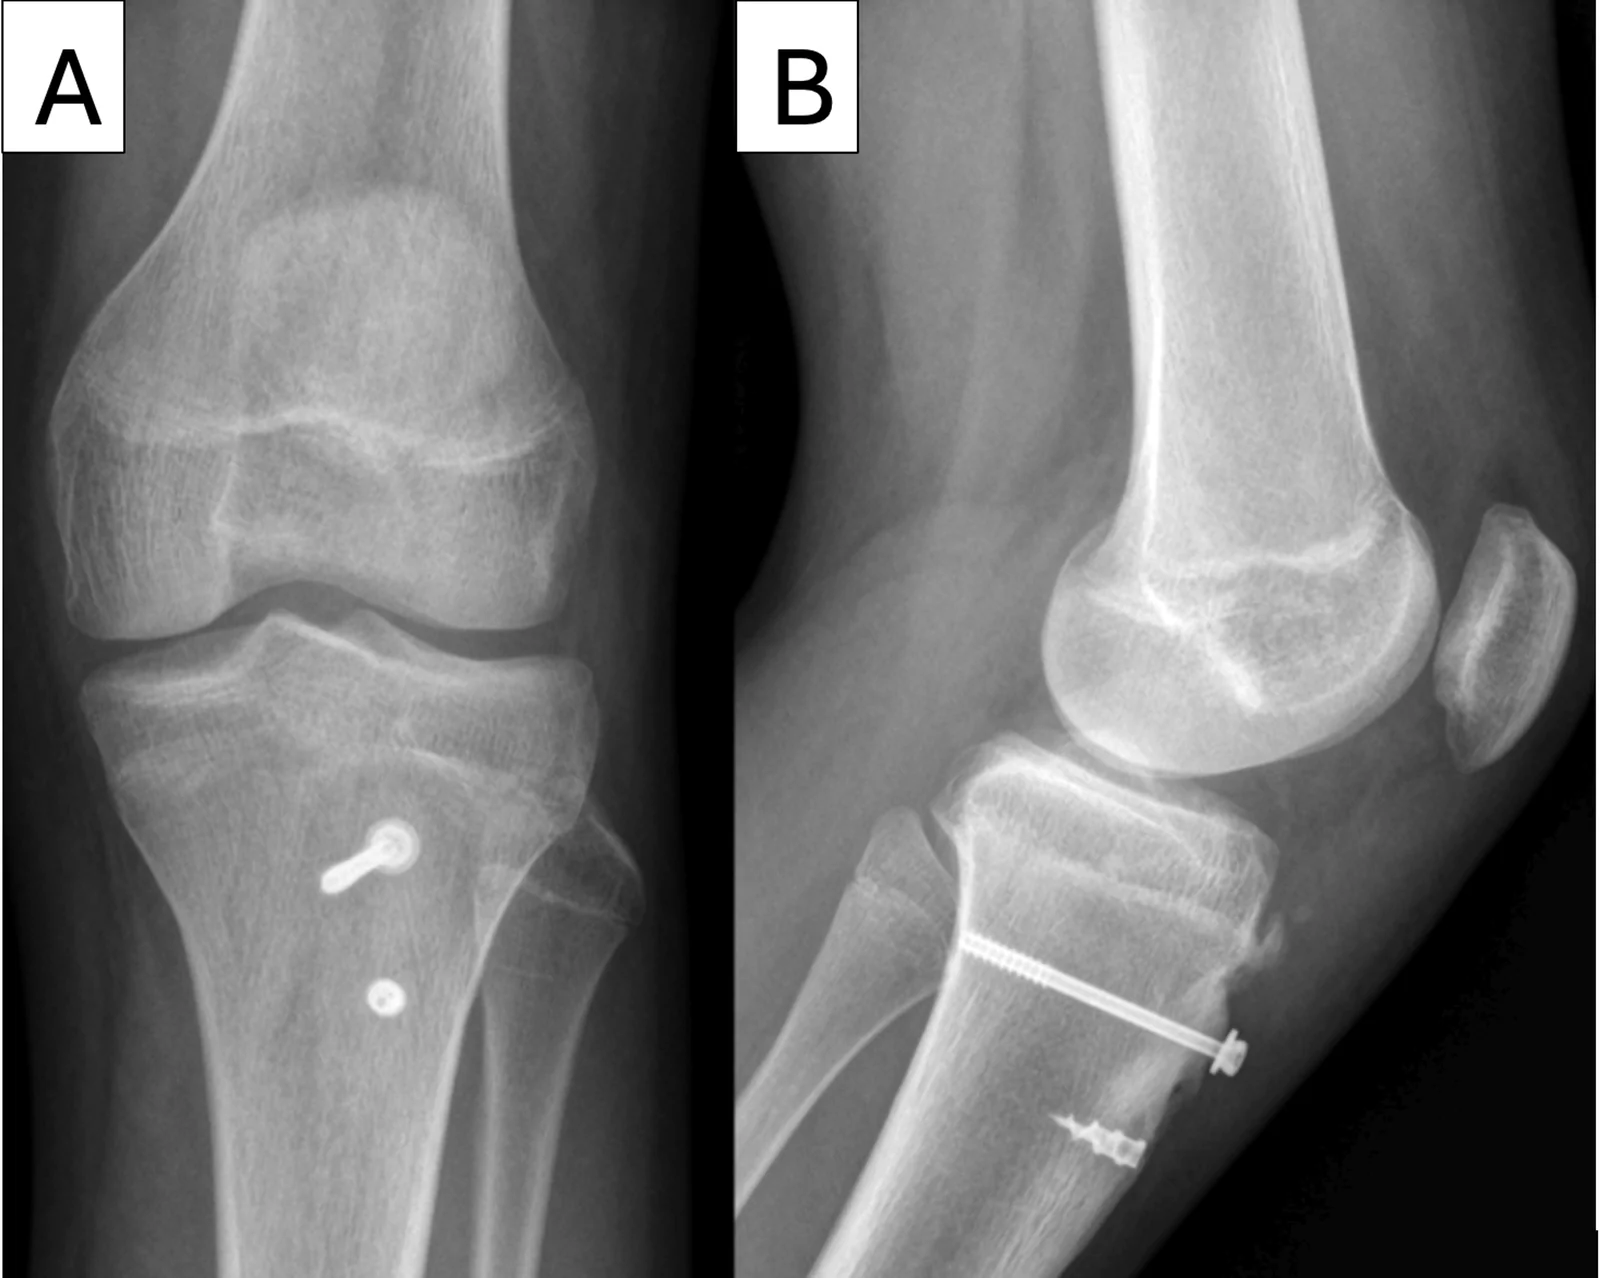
Postoperative radiographs of case 3. (A) Anteroposterior and (B) lateral radiographs obtained six months postoperatively demonstrating maintained reduction, stable fixation, and fracture union following open reduction and internal fixation of the tibial tubercle with concomitant patellar tendon repair.
